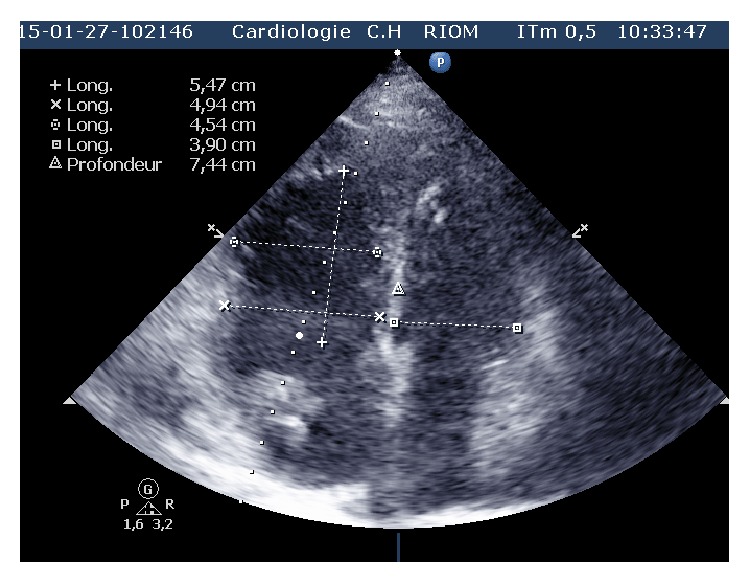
Apical four chamber view showing right ventricular dimensions (basal right ventricular diameter = 4.94 cm, mid-right ventricular diameter = 4.54 cm).
